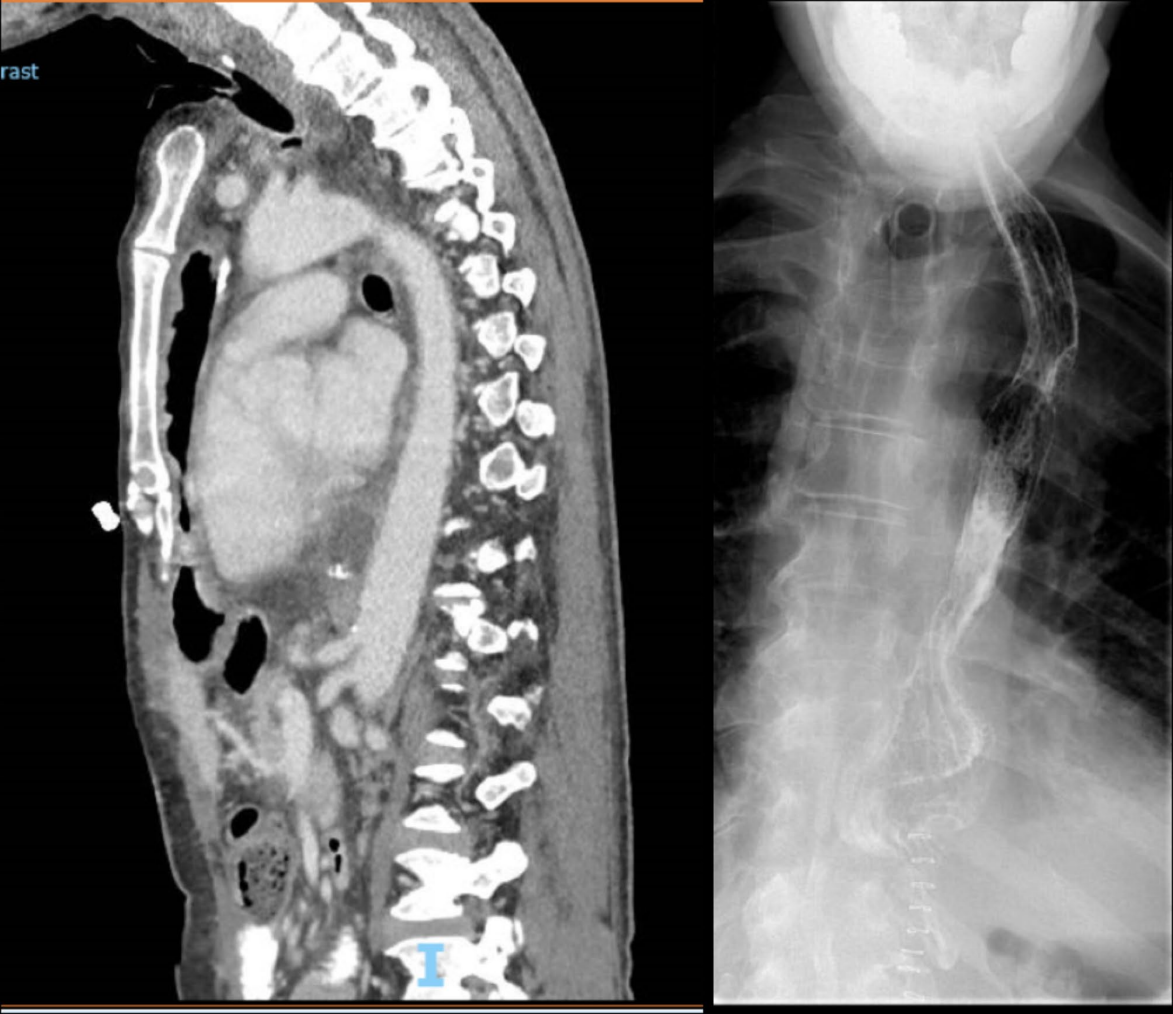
Post-operative radiological images. CT scan (left) showing retrosternal passage of reconstructed gastric tube conduit. Barium swallow (right) showing no leak from superior pharyngo-gastric anastomosis
